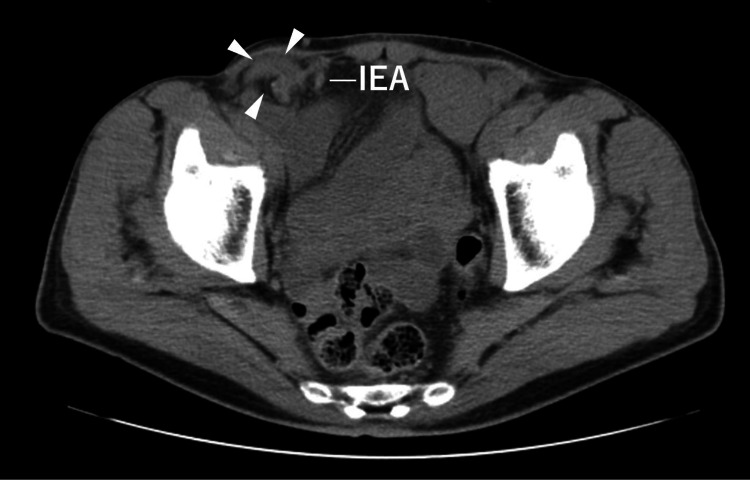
Preoperative plain abdominal computed tomography scan The small intestine (arrowheads) prolapses into the right inguinal hernia from outside the right inferior epigastric artery IEA, inferior epigastric artery
